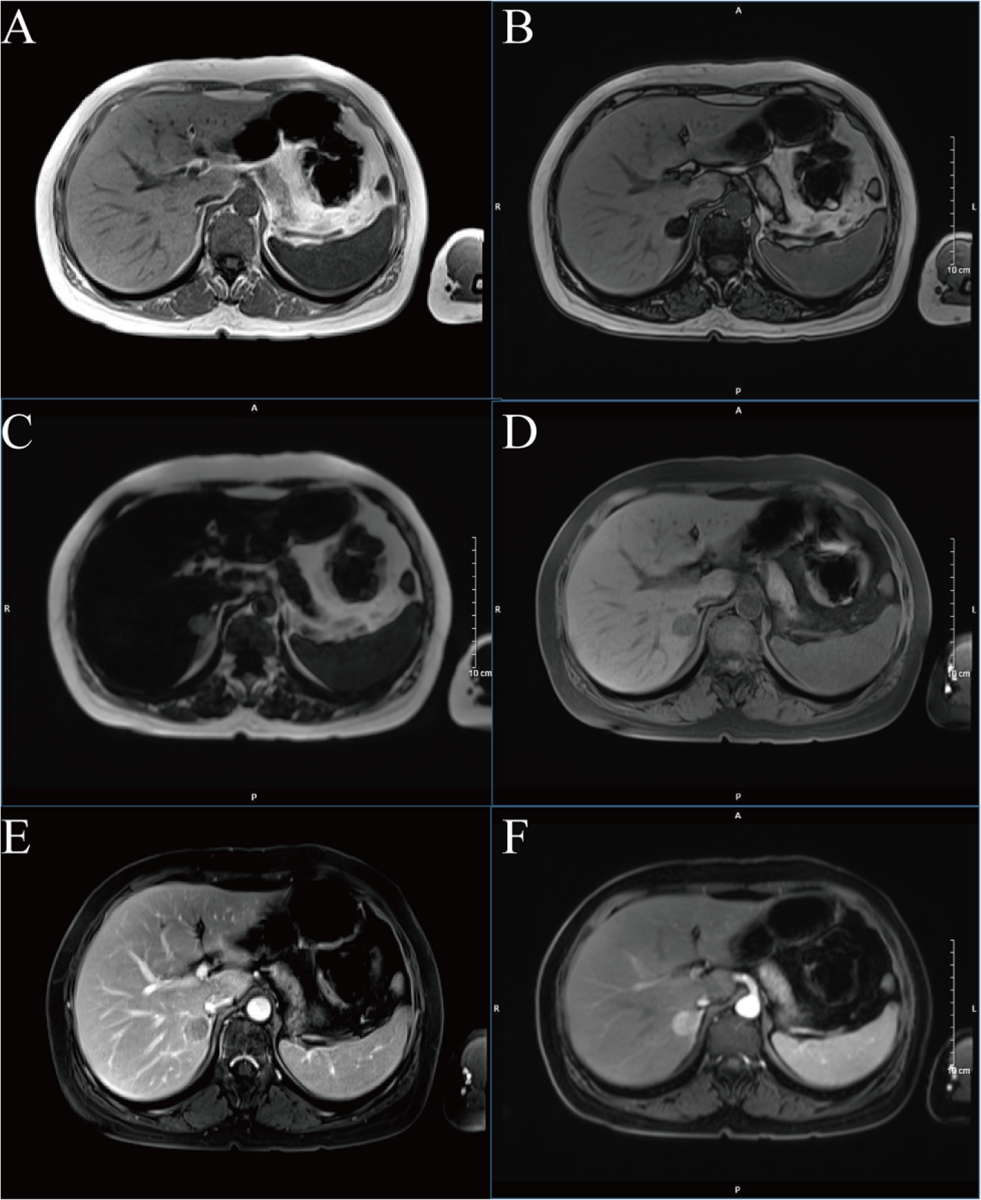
Enhanced magnetic resonance imaging (MRI) uncovered an abnormal signal of the nodule (20 mm × 18 mm with clear boundary) at the S7 section of the liver. T1WI presented equisignals, and the antiphase was considerably lower than the in-phase. T2WI and T2WI + FS presented slightly higher signals, DWI presented a high signal, and the ADC presented a slightly lower signal. These signals were considerably enhanced during enhanced arterial phase scanning but disappeared during the venous portal and delayed stages. All signals detected were located lower than the liver parenchyma
